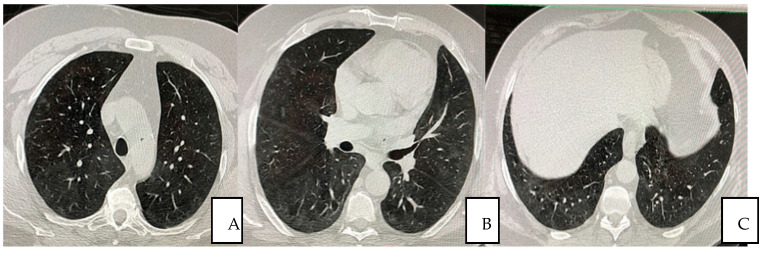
(A–C) Pulmonary HRCT scan obtained on day 35 from symptom onset (23 December 2020) shows almost complete resolution of the initial presentation.
